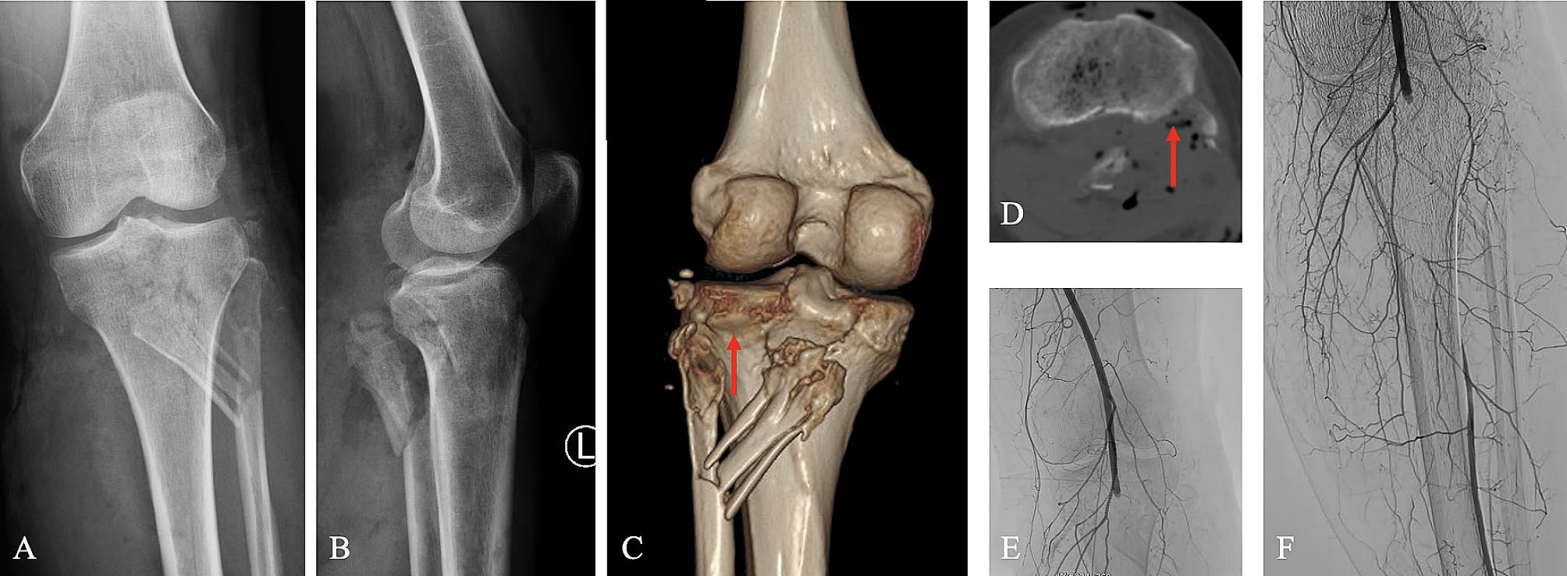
Traumatic proximal tibiofibular fracture and dislocation (posteromedial dislocation). AP view (A), lateral view (B), Axial CT (D) and 3D reconstruction (C) shows proximal fibula fracture with posteromedial dislocation of fibular head (red arrow indicates the tibial articular surface of proximal tibiofibular joint). Angiography (E, F) indicates popliteal artery injury
